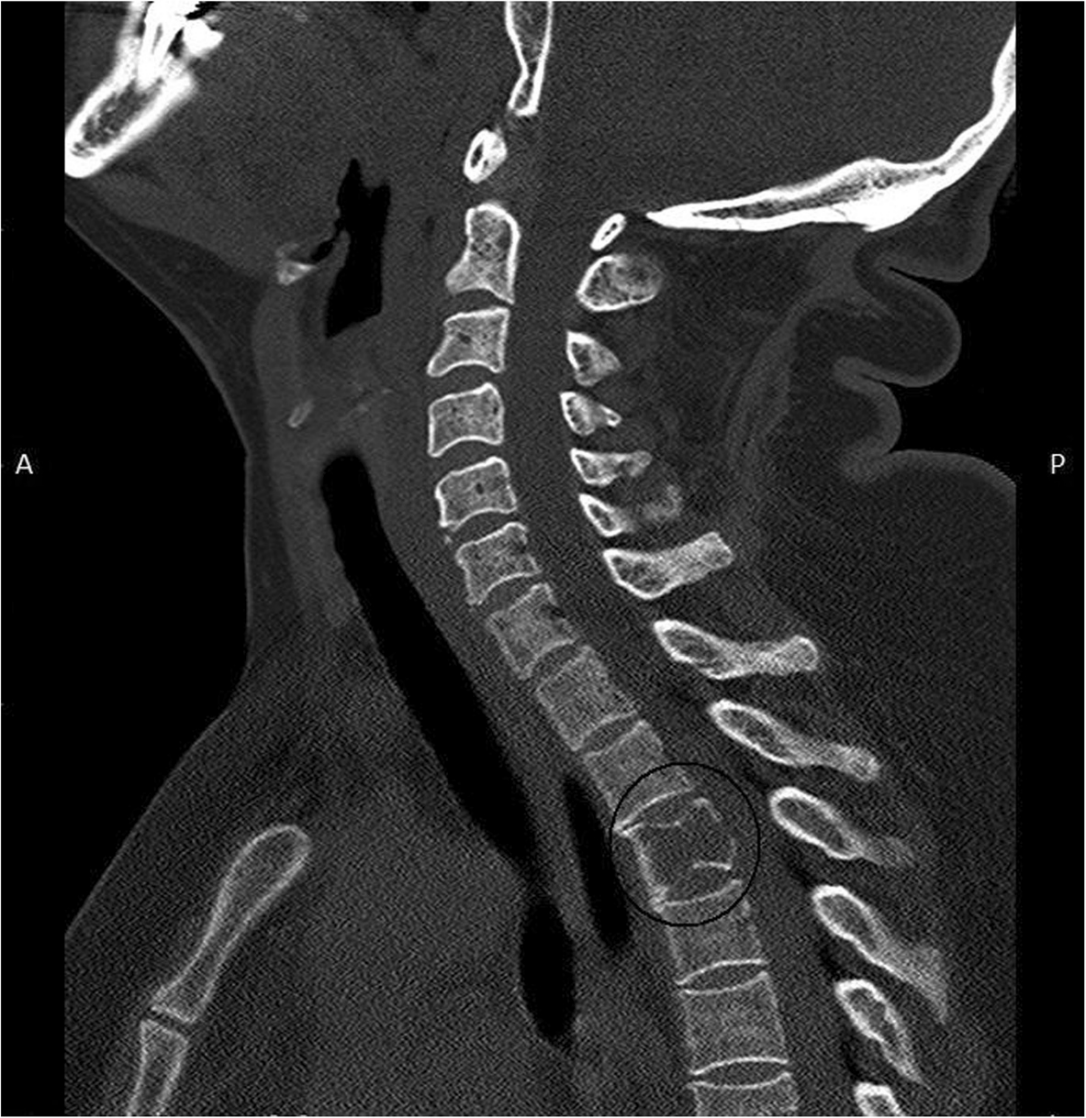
Computed tomography (sagittal reconstruction) showing metastatic mass from HCC infiltrating body of third dorsal vertebra (circle)*Lymph node metastases (9 cases)*Six cases were located in the abdominal lymph nodes and 3 were situated in the retroperitoneal, inguinal and supraclavicular lymph nodes. Guided needle or excisional biopsies from these sites were performed.*Soft tissue metastases (7 cases)*These metastases were located in the anterior chest wall (4 cases), shoulder (1 case), back, deep to angle of scapula (1 case) and forearm (1 case). CT scan revealed a large soft tissue mass with no underlying bone destruction. Needle biopsies were taken for pathological assessment.*Omental metastases (7 cases)*The main complaint of these cases was abdominal pain, CT scan showed hypoechoic solitary mass in 2 patients and multiple nodules in 5 patients.*Adrenal gland metastases (2 cases)*The patients presented with lumbar pain. Abdominal ultrasound detected a retroperitoneal mass close to the kidney. CT scan with contrast was done and revealed an enhancing adrenal mass from which needle biopsy was taken.*Brain metastases (2 cases)*Both patients complained of headache and vomiting, CT scan showed a space occupying lesion in the left parietal lobe and right fronto-temporal area with homogeneous enhancement. Craniotomy was done and the tumor was excised.*Maxillary sinus metastases (2 cases)*One of the two patients presented with severe bleeding after tooth extraction. The other had felt pain on occlusion and movement of the right upper molar, CT examination of both cases revealed soft tissue density infiltrating the adjacent bones and muscles.*Skin metastases (1 case)*One patient presented with a cutaneous non ulcerating nodule on his hand. Radiography and CT scan of the hand suggested a subcutaneous metastasis.
